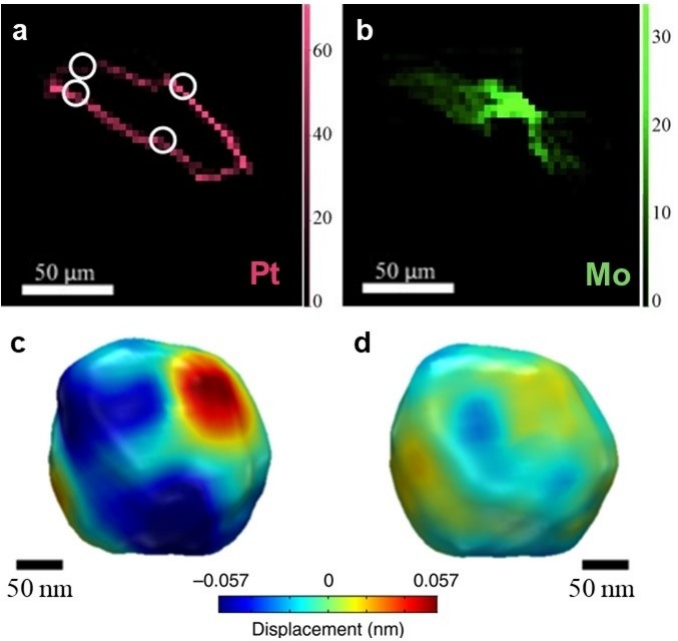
a),b) μ‐XRF‐CT cross‐section of a single Mo‐promoted Pt/C catalyst particle, showing a) Pt and b) Mo elemental maps during liquid‐phase hydrogenation of nitrobenzene. c),d) Bragg CDI‐reconstructed 3D images showing the displacement distribution (lattice distortion) on a single 220 nm Pt NP at the c) start and d) end of methane oxidation. Adapted from Refs. 47, 69.
